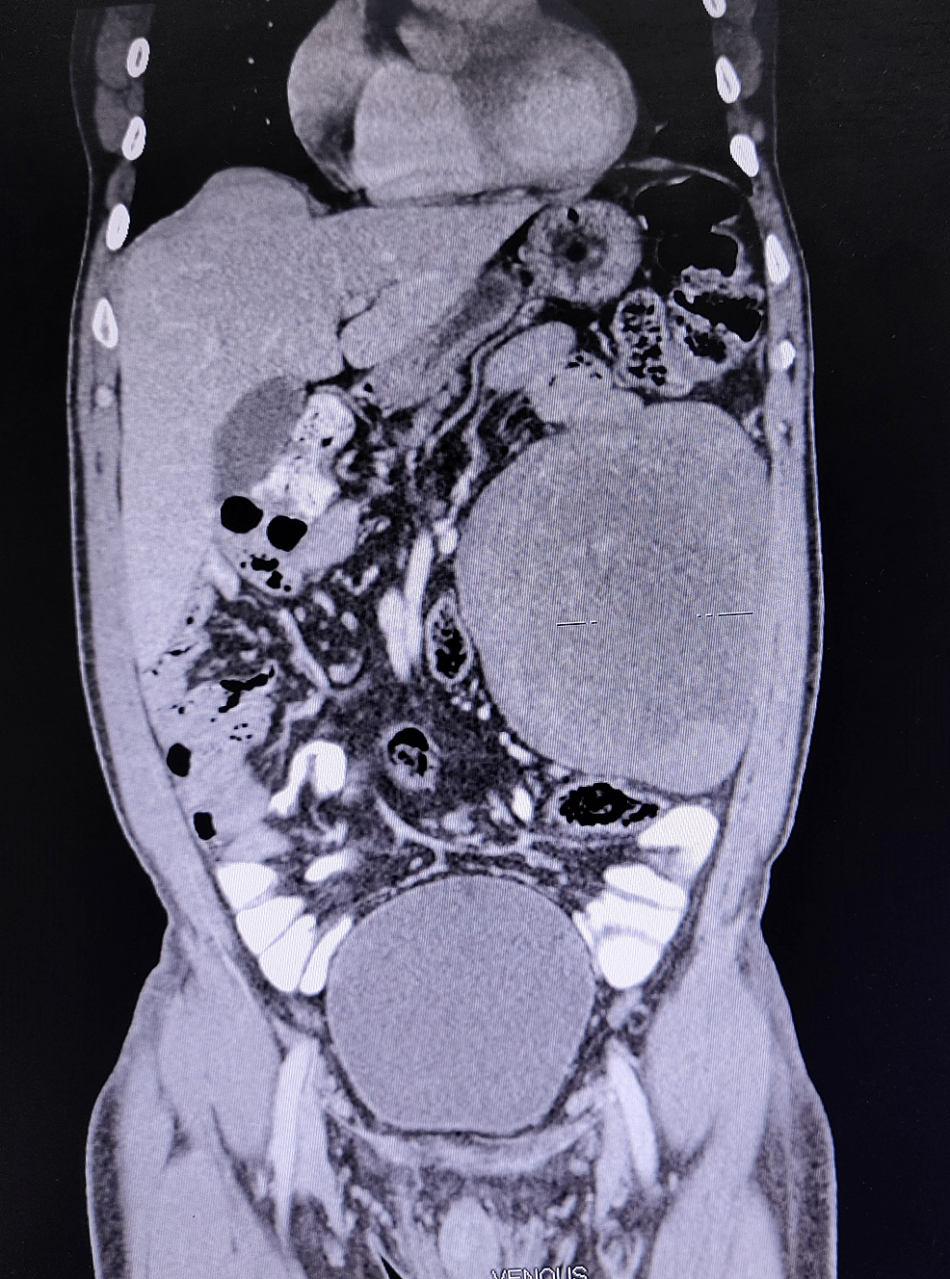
Coronal section of CT abdomen showing the tumour and its relation with the surrounding structures
